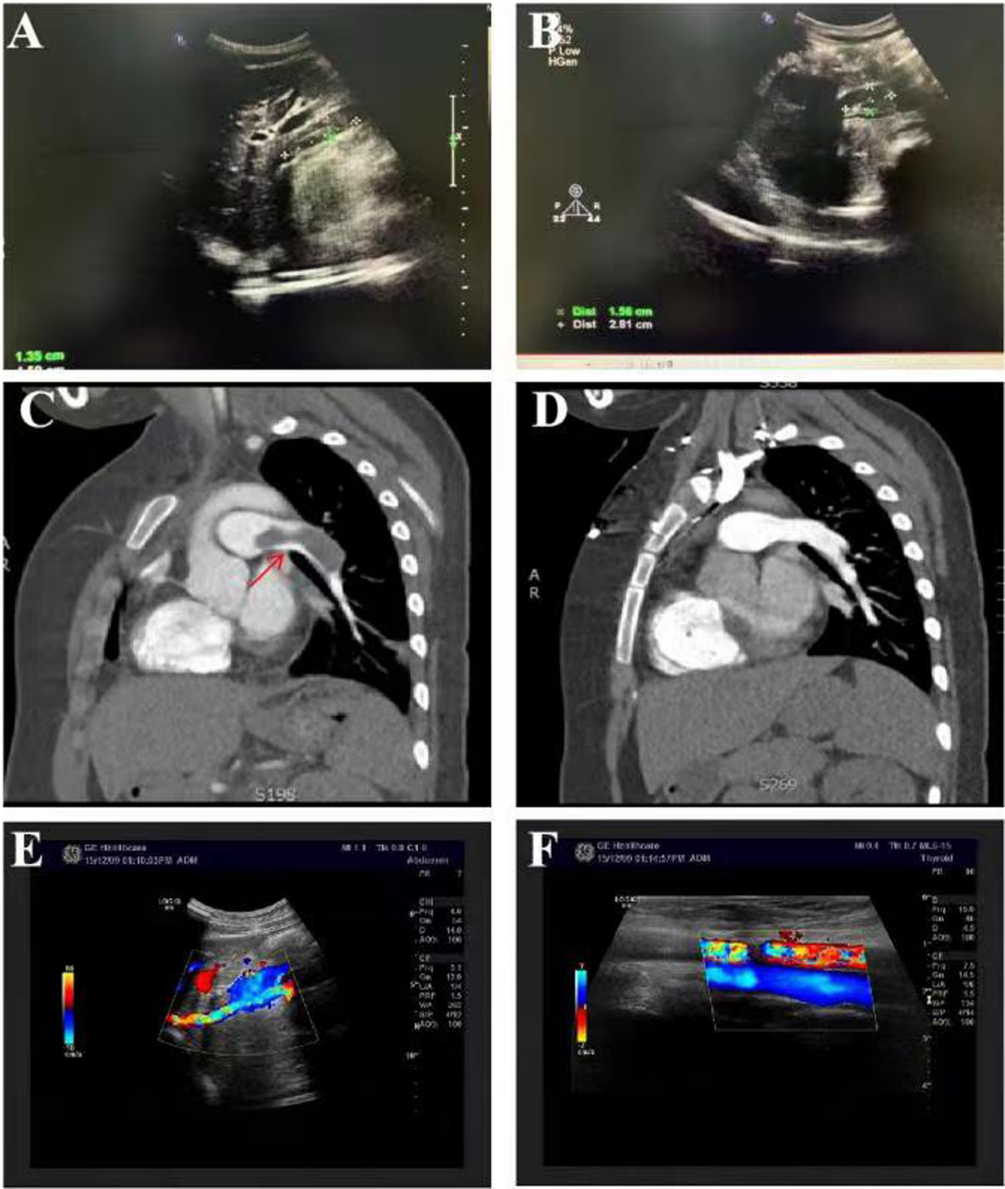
Clinical features of the current patient.* Among auxiliary examinations, color Doppler ultrasound showed hypoechoic masses in the wall of the inferior vena cava (A), and of the right renal vein (B), considering the possibility of thrombosis. Pulmonary artery CT angiography (CTA) showed embolisms of the distal main pulmonary artery, left pulmonary artery trunk and its intrapulmonary branches, and the right lower pulmonary artery (C), considering the possibility of ischemic infarction. The thrombi disappeared after effective treatment (D). Vascular B-ultrasound showed bilateral popliteal vein blood flow, and bilateral mural thrombosis was considered (E-F).
